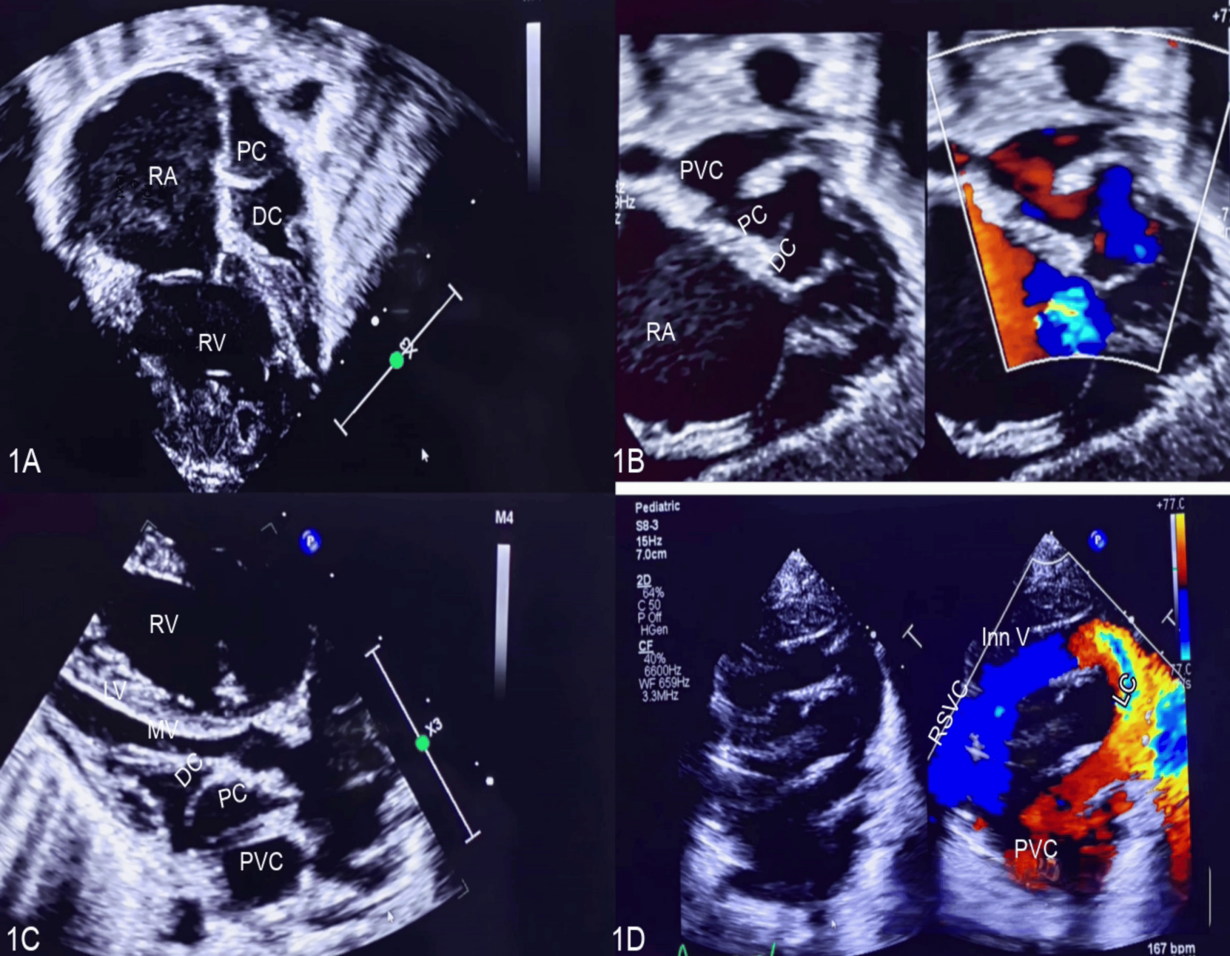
Transthoracic echocardiographic findings of the cor triatriatum and levoatriocardinal vein 1A: The four-chamber view shows that the left atrium is divided into the proximal chamber (PC) and distal chamber (DC) by a cor triatriatum membrane with a significant dilatation of the right atrium (RA) and right ventricle (RV). 1B: The colour compare subcostal image shows pulmonary venous confluence (PVC), proximal and distal chambers. IC: The parasternal long-axis view shows the squashed left ventricle due to pulmonary hypertension. 1D: The levoatriocardinal vein (LC) connects the pulmonary venous confluence to the left innominate vein (inn V). RSVC, right superior venacava
